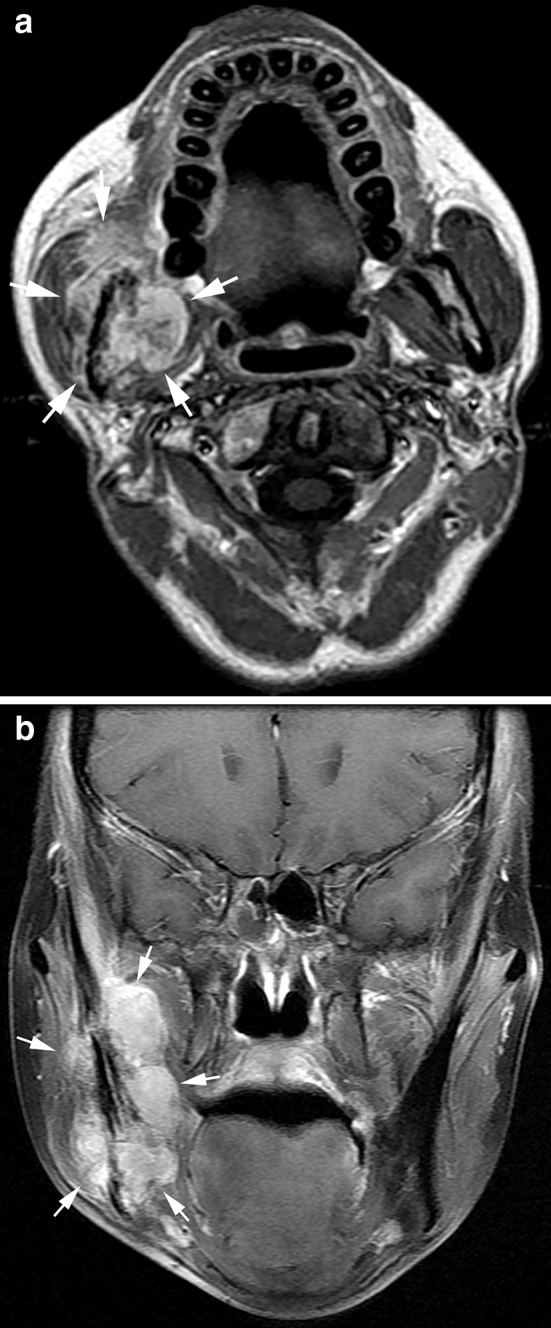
Radiation-induced fibrosarcoma 5 years after radiotherapy of a 35-year-old woman. Axial T1-weighted contrast-enhanced image (a) and coronal fat-suppressed T1-weighted contrast-enhanced image (b) showed a large heterogeneously marked enhanced tumor of the right mandibular ramus with bone destruction and wide invasion into the muscles (*arrows*)
